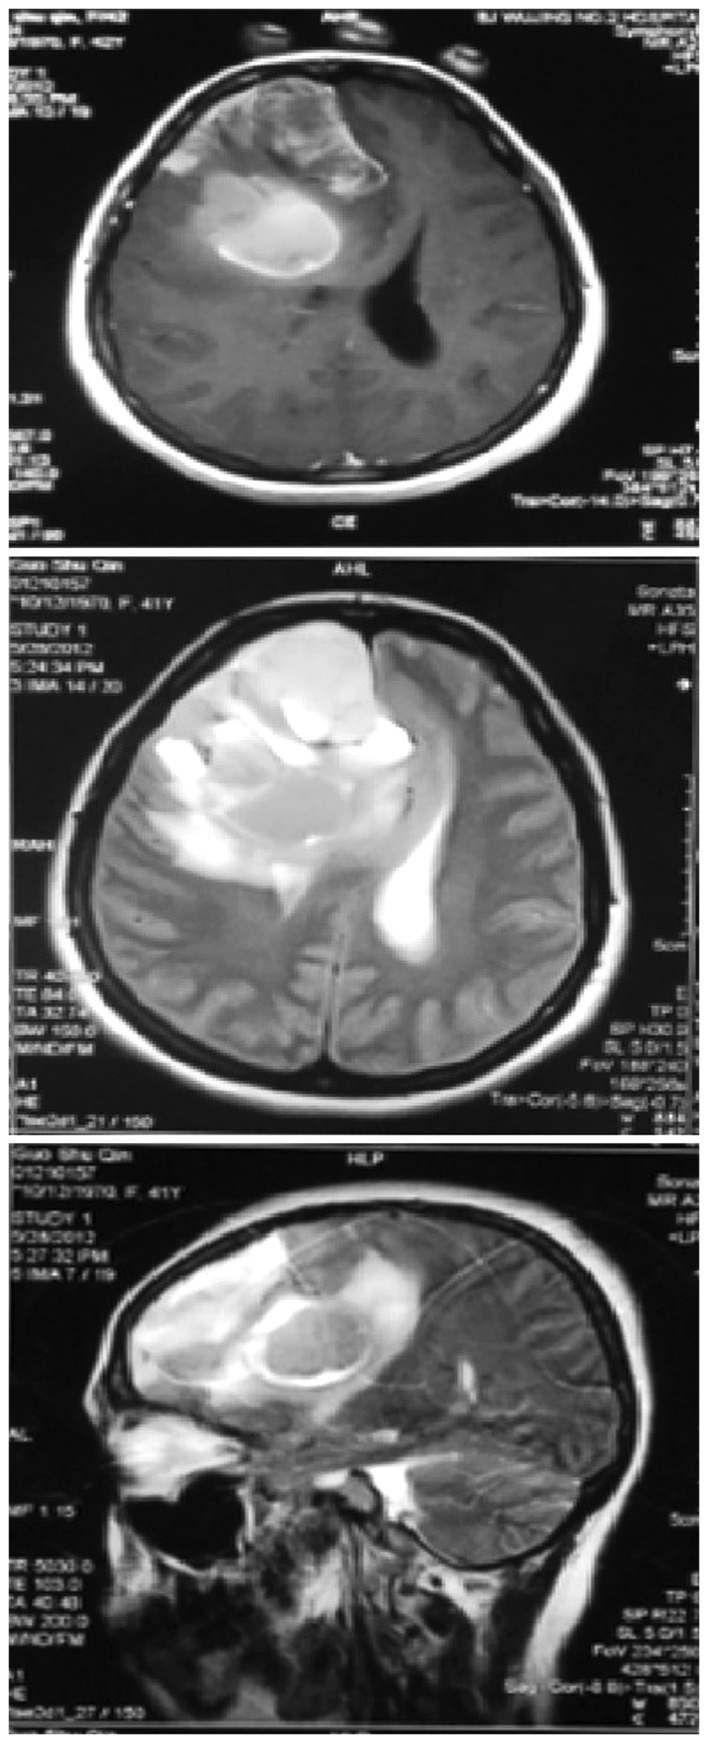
Images from a magnetic resonance imaging (MRI) scan of case 2.
